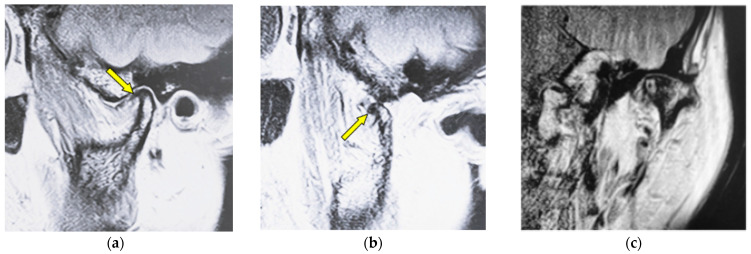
MR image (proton-density-weighted) of a 53-year-old woman (disc displacement without reduction, left disc): (a) sagittal oblique cross-section imaging in the mouth-closing position; (b) sagittal oblique cross-section imaging in the mouth-opening position; (c) coronal oblique cross-section imaging in the mouth-closing position. The arrow shows the part with an osteophyte.
